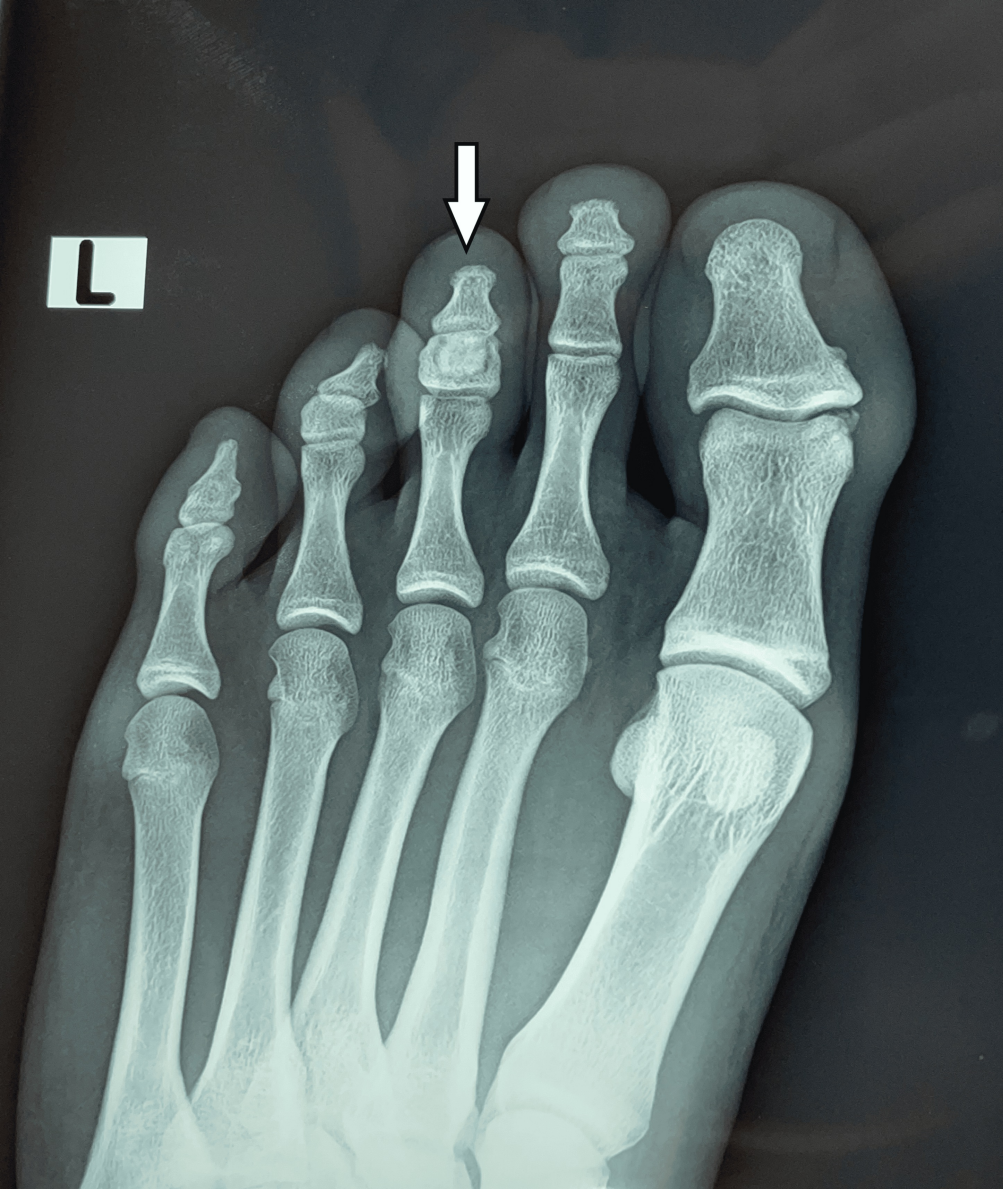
AP X-ray showing a central sclerotic lesion in the middle phalanx of the third toe, surrounded by a thin radiolucent zone
